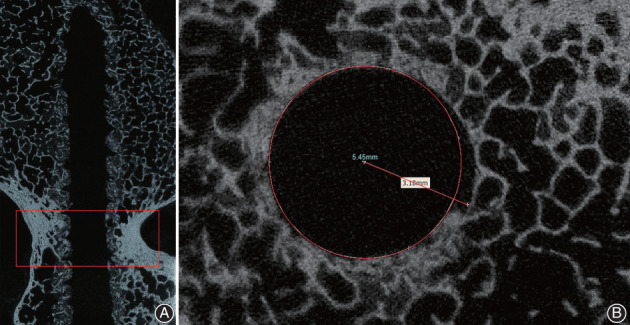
(A) Micro‐CT image of surrounding bone structure of screw insertion area of pedicle zone; and (B) the semidiameter was defined as distance between the thread peek point and the center of the largest circle of insertion cross area.
